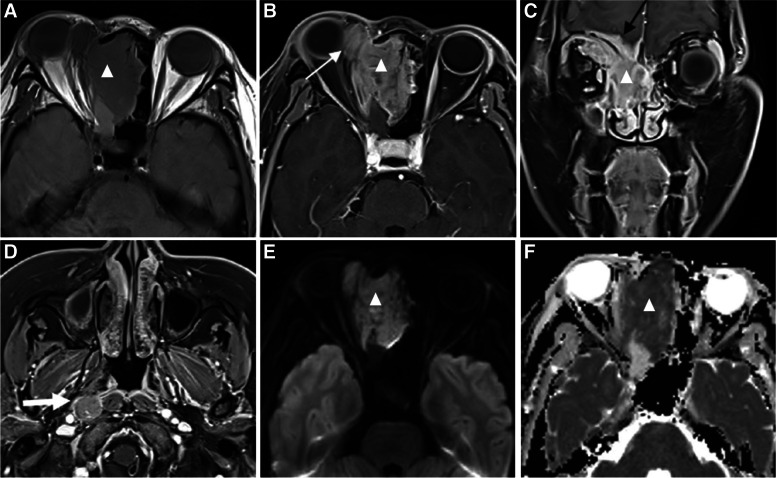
A 39-year-old female patient with ARMS. a T1-weighted and b, c, d T1-weighted fat-suppressed postcontrast images show an irregular soft tissue mass (triangle) in the right ethmoid sinuses with right retropharyngeal lymph node metastasis (thick white arrow). The size of the mass is 4.6 × 3.6 × 4.2 cm^3^ and the margin is ill-defined. After contrast enhancement, the mass is enhanced markedly and heterogeneously. Orbit involvement (thin white arrow) and skull involvement (thin black arrow) can be seen. e DWI shows slightly high signal in the mass (triangle). f ADC map shows a low signal (triangle) with measured ADC of 0.863 × 10^–3^ mm^2^/s
